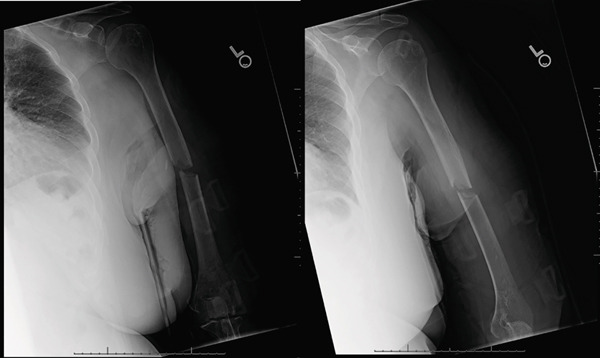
AP and lateral radiographs of the left humerus taken at the 2‐week follow‐up visit in the Sarmiento brace. Alignment is improved compared with initial injury films with some mild distraction. No sign of callous formation.
